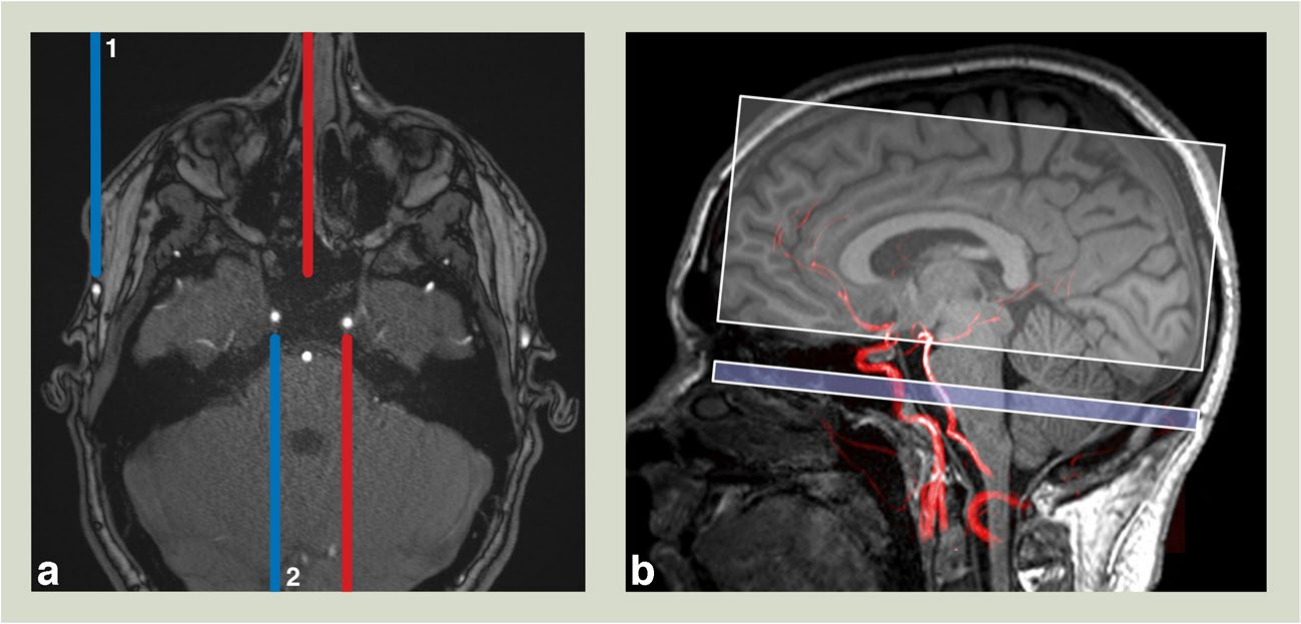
Axial MRI image with an exemplary vessel-encoded arterial spin labeling scheme (a). The blue and red lines depict the locations that are placed in contrast. Top (1): tagging of the right superficial temporal artery and a point midway between both internal carotid arteries. Bottom (2): tagging of both internal carotid arteries. Sagittal MRI image with time-of-flight maximum intensity projection (b) depicting the labeling position (slim lower bar) and the upstream brain volume (thick upper bar)
